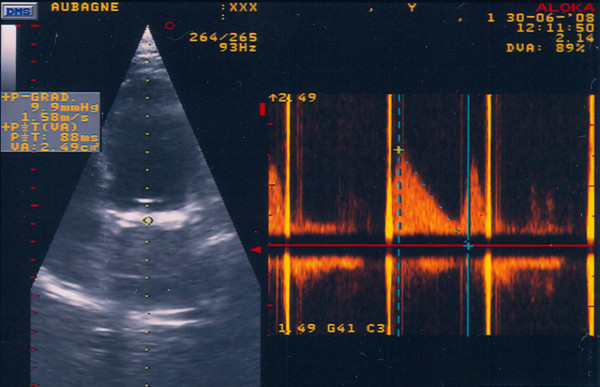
Recording of the transprothetic mitral inflow by continuous wave Doppler in a 4-chamber apical view. The peak early diastolic velocity was 158 cm/s, the mean gradient 3.5 mmHg and PHT 88 ms.
